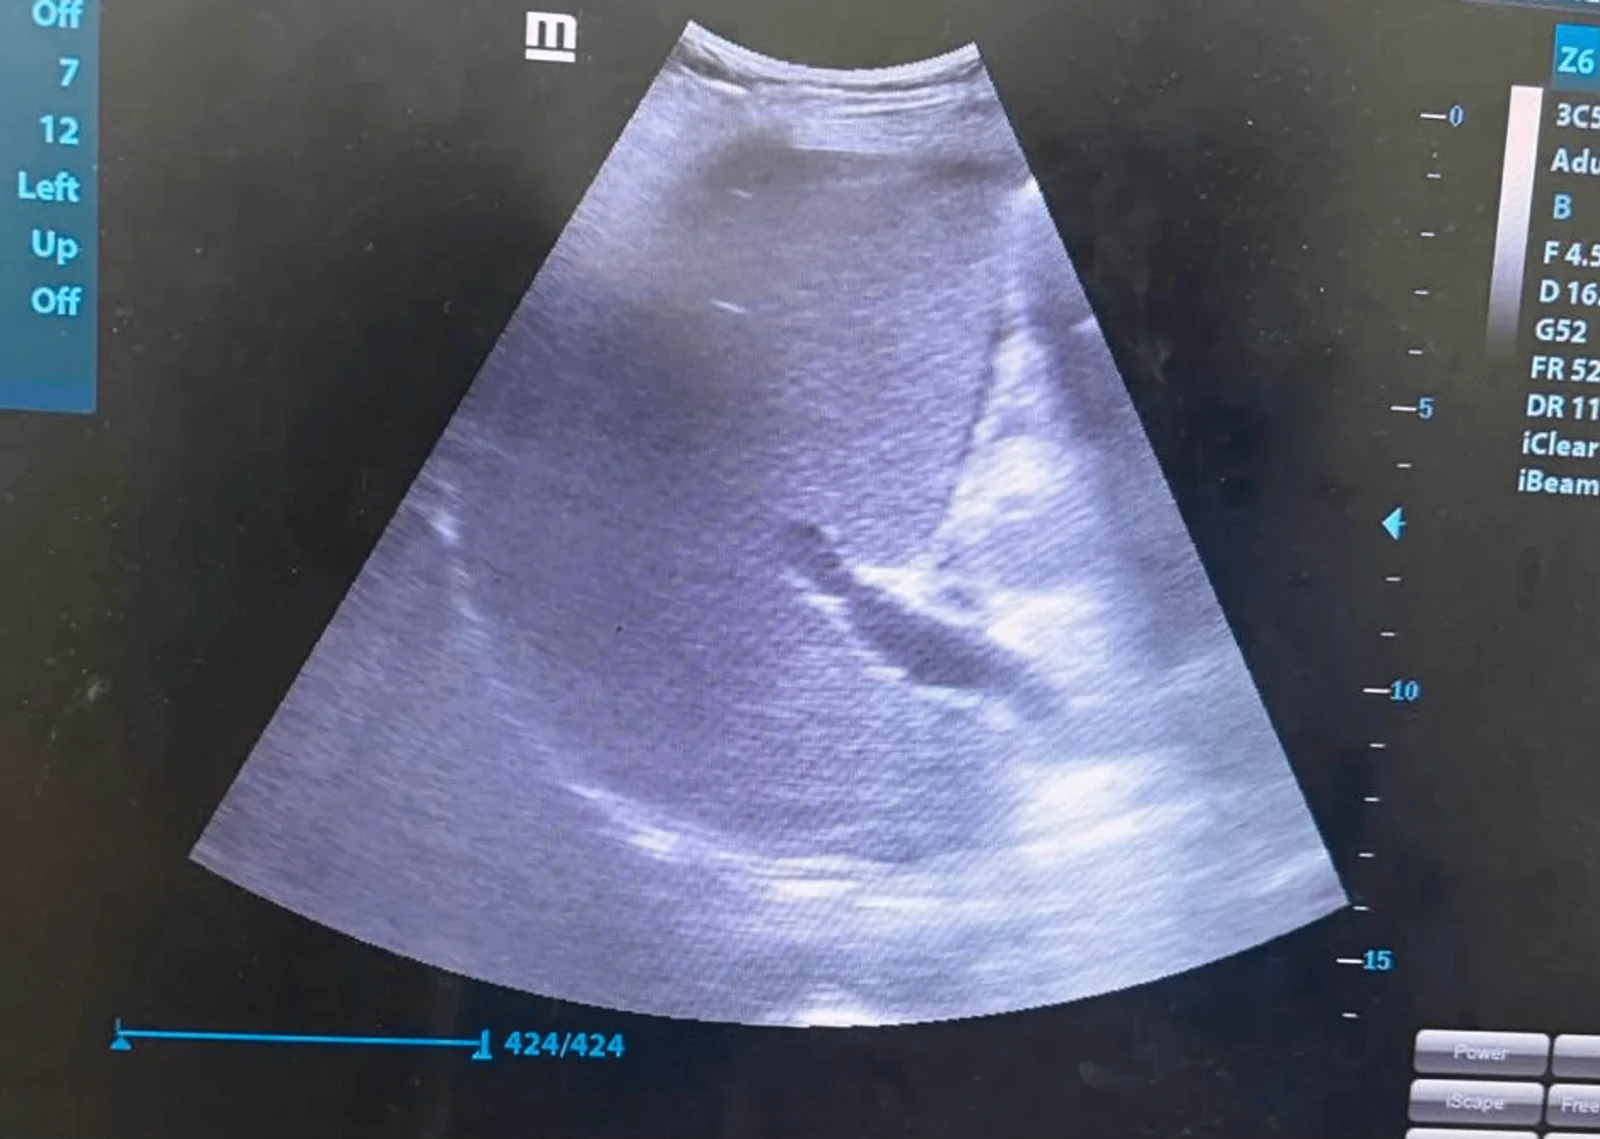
Abdominal ultrasound showing free fluid in the subhepatic region
